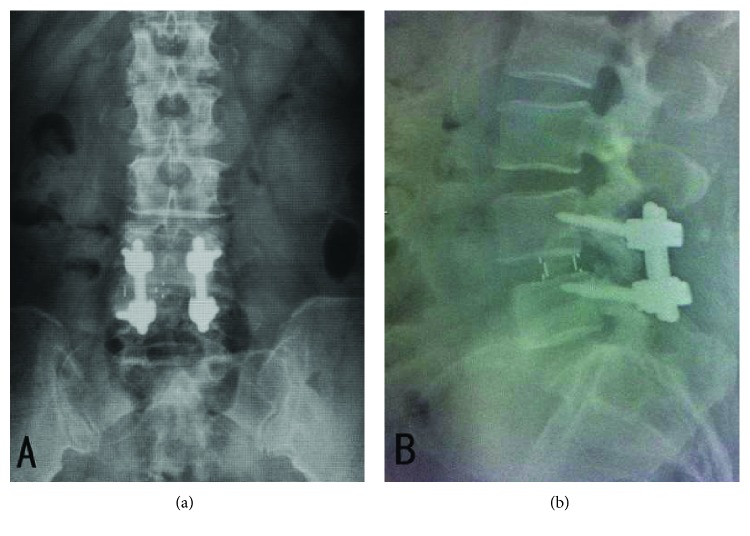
Three months postoperative plain films of lumbar spine AP and lateral (a and b) showed the acceptable position of MIS-OLIF PEEK cage.
